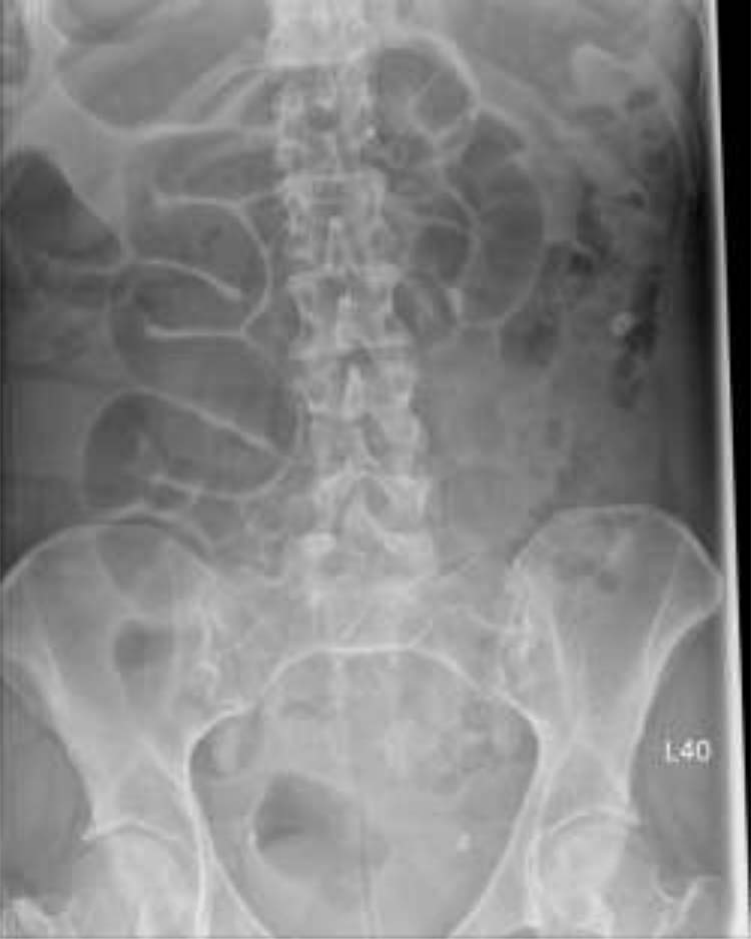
Abdominal X-ray shows twisted dilated small bowel loops.
